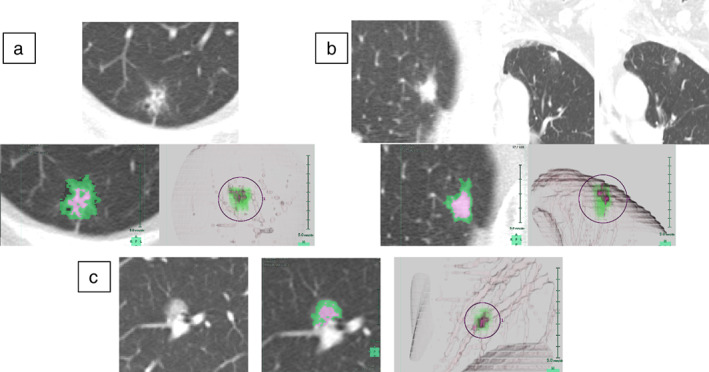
Computed tomography (CT) images and 3D imaging analysis of characteristic cases. A solid component is present in the spots (a). It is difficult to visualize the maximum solid site and ground‐glass opacities (GGO) in a simple single plane image (b). The lesion was a pure GGO on CT but the Synapse Vincent software program recognized a slight difference in the gradation of the CT value and it was judged that there was a solid component (c)
